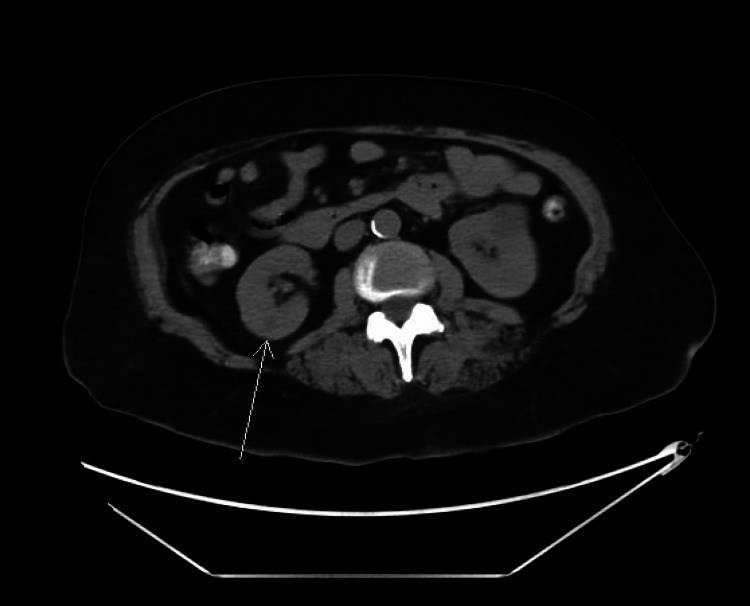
CT of the abdomen and pelvis demonstrating indeterminate small structure in the kidney CT: computed tomography
